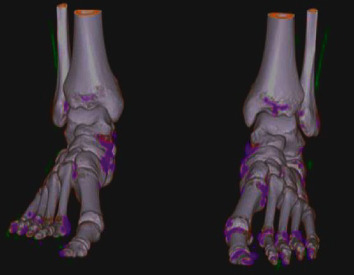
Dual-energy CT demonstrating sodium monourate deposits in both feet.
